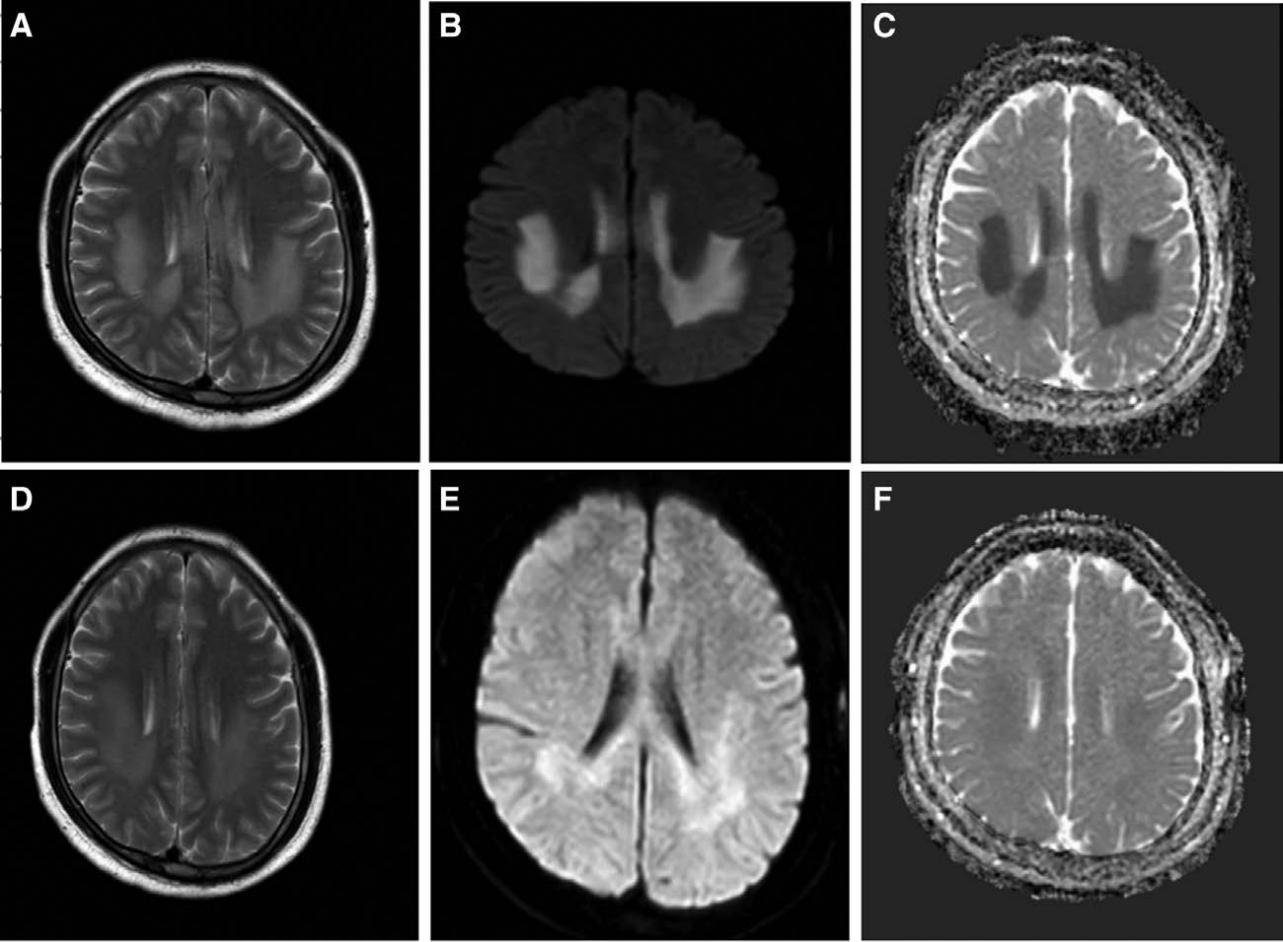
MRI imaging at 6 hours following the onset of symptoms showing increased signals on T2-images in the SCC and frontal-parietal subcortex of both cerebral hemispheres (A). Abnormal signals were also found at DWI (B) and ADC images (C) in the same area. MRI imaging at 8 days later exhibited reduced abnormal signals in T2 (D), DWI (E), and ADC (F) in the same area. ADC = apparent diffusion coefficient, DWI = diffusion-weighted imaging, MRI = magnetic resonance imaging, SCC = splenium of the corpus callosum.
